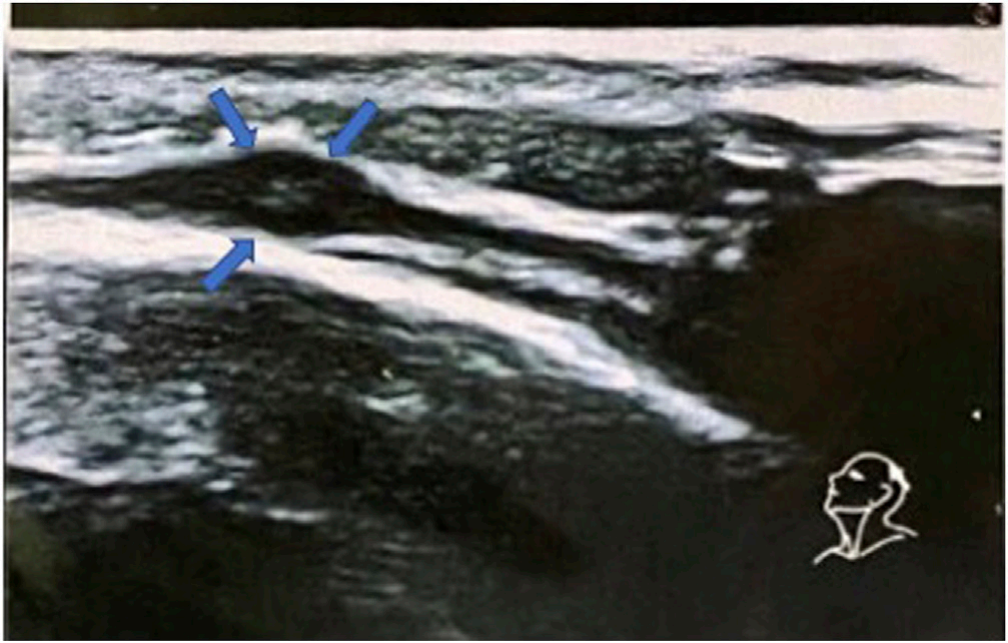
Ultrasound images of scalp nodules showing fibrotic and granulomatous characteristics.
